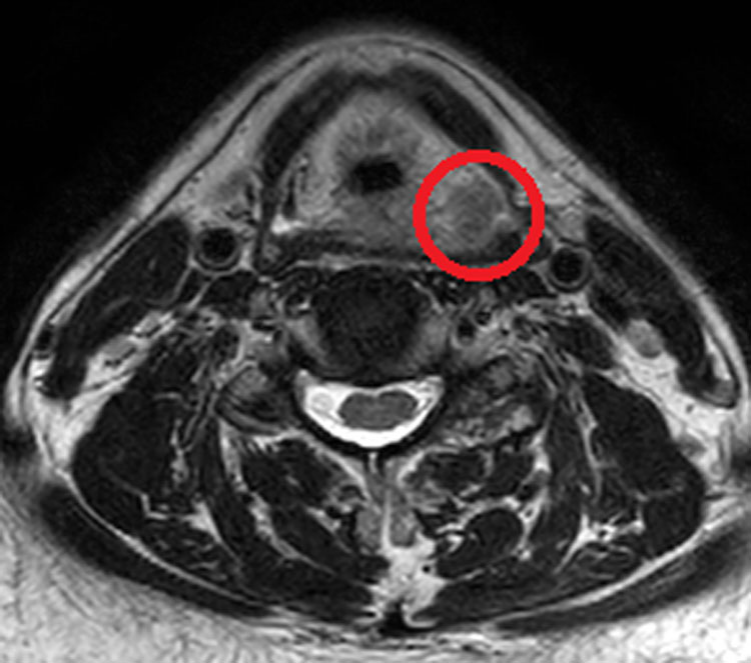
The patient's head MRI showed a nodule (red oval) behind the trachea.
